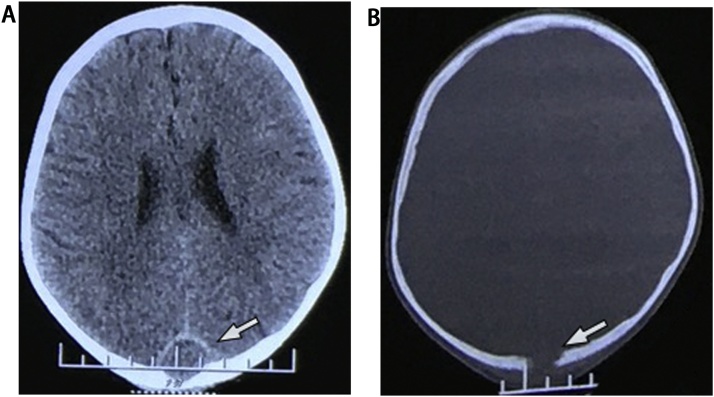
On CT scan, an occipital well-defined isodensity round mass had a thin enhanced peripheral rim, invaded skull bone, and superior sagittal sinus.
